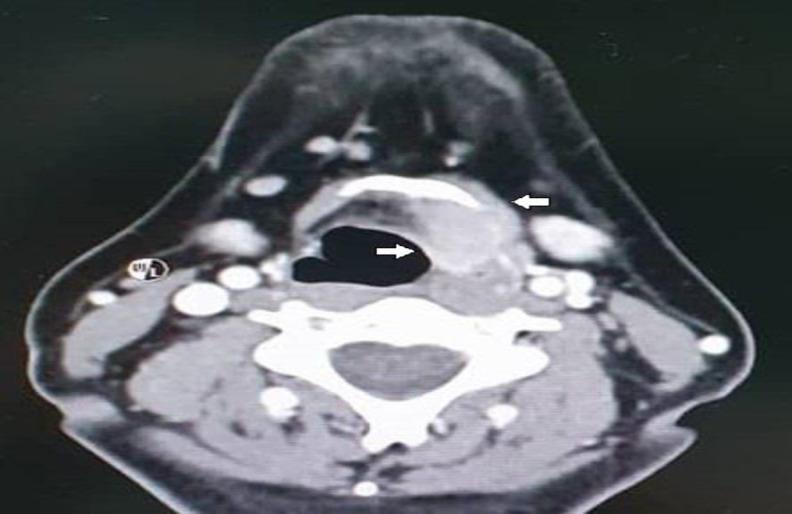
Computed tomographic scan contrast of the neck showing homogenously enhancing lesion at left supraglottic region measuring 2.3 x 1.7 x 2.5cm (white arrows). This lesion is causing widening of the paraglottic fat with medialisation of mucosal space
